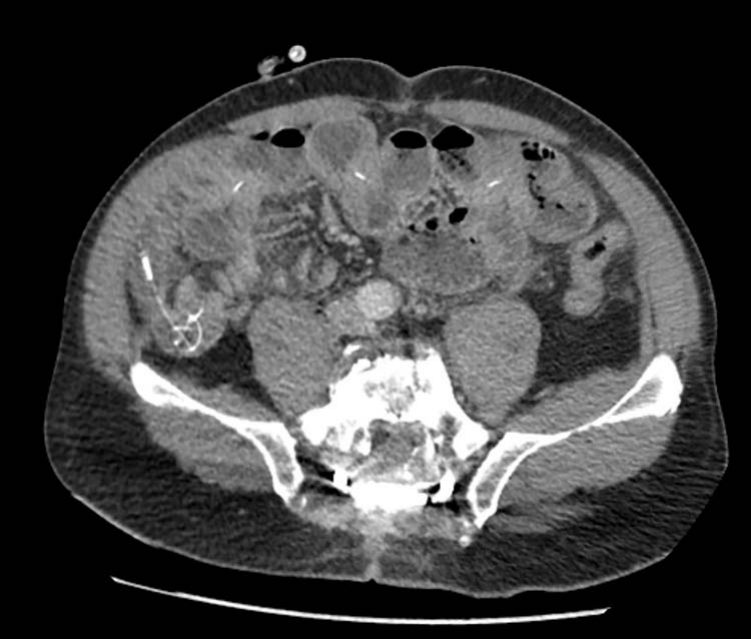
Preoperative CT scan showing intestinal occlusion and a knot formation.
